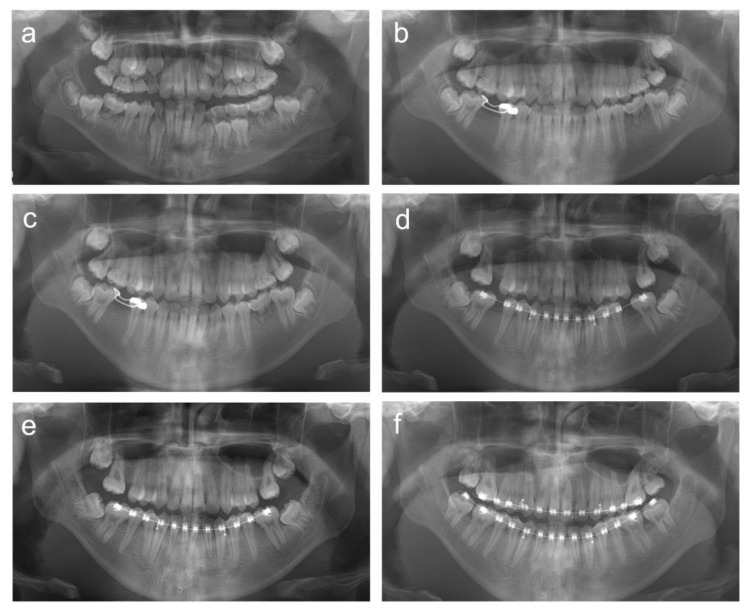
Panoramic radiographs of patient 4. (a) Panoramic radiograph at age 11 years 8 months. (b) Panoramic radiograph at age 15 years 10 months. (c) Panoramic radiograph at age 16 years 5 months. (d) Panoramic radiograph at age 17 years 5 months. (e) Panoramic radiograph at age 17 years 10 months. (f) Panoramic radiograph at age 19 years 1 month.
